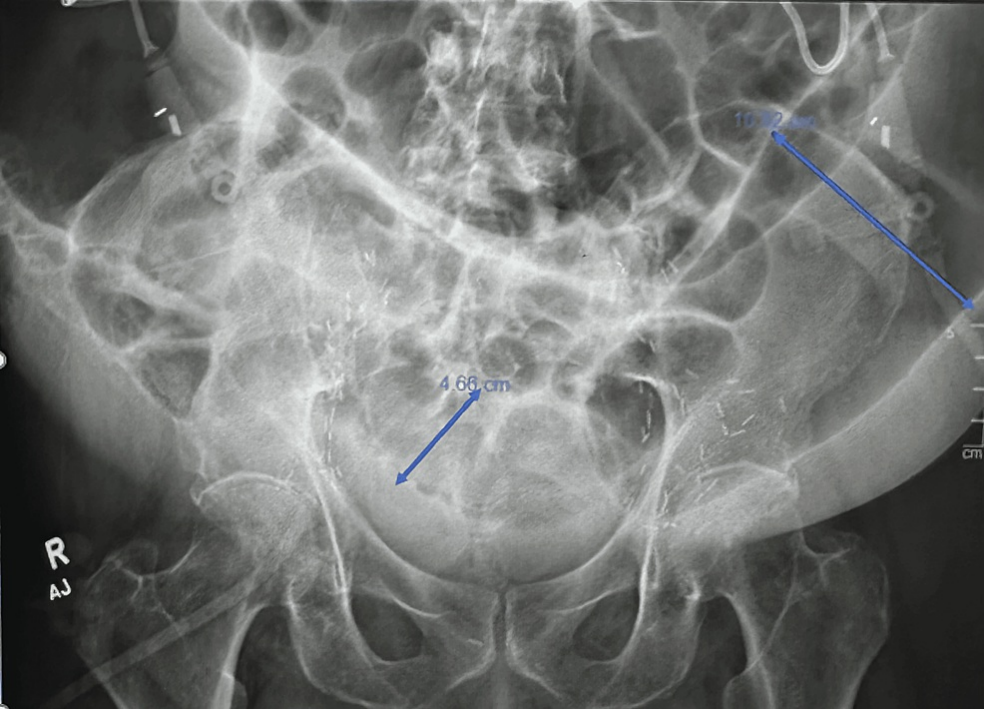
Abdominal X-ray showing severe colonic distention
